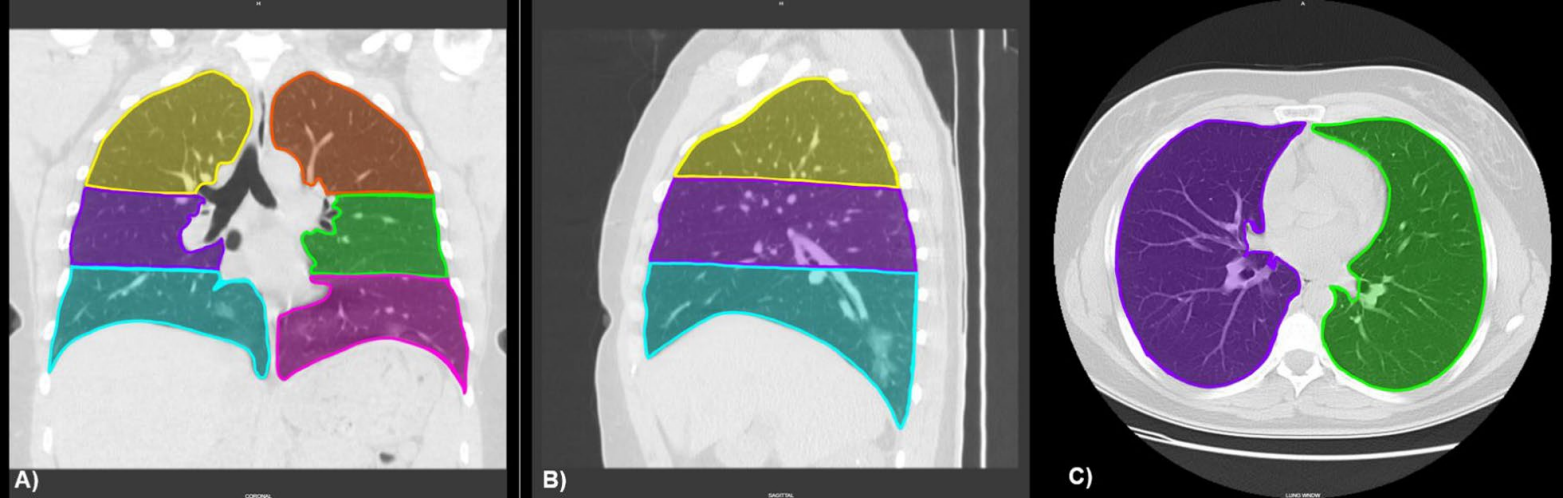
Ichikado pulmonary zones. The upper zone is defined as the lung parenchyma above the carina, the middle zone as the area between the carina and the pulmonary vein, and the lower zone as the infrapulmonary vein area. A Coronal lung window of CT chest showing all 6 Ichikado pulmonary zones. B Sagittal lung window of CT chest showing right lung Ichikado areas. C Axial lung window of CT chest showing right and left middle zones. This example shows images of a 34-year-old lady with coronavirus disease 2019
